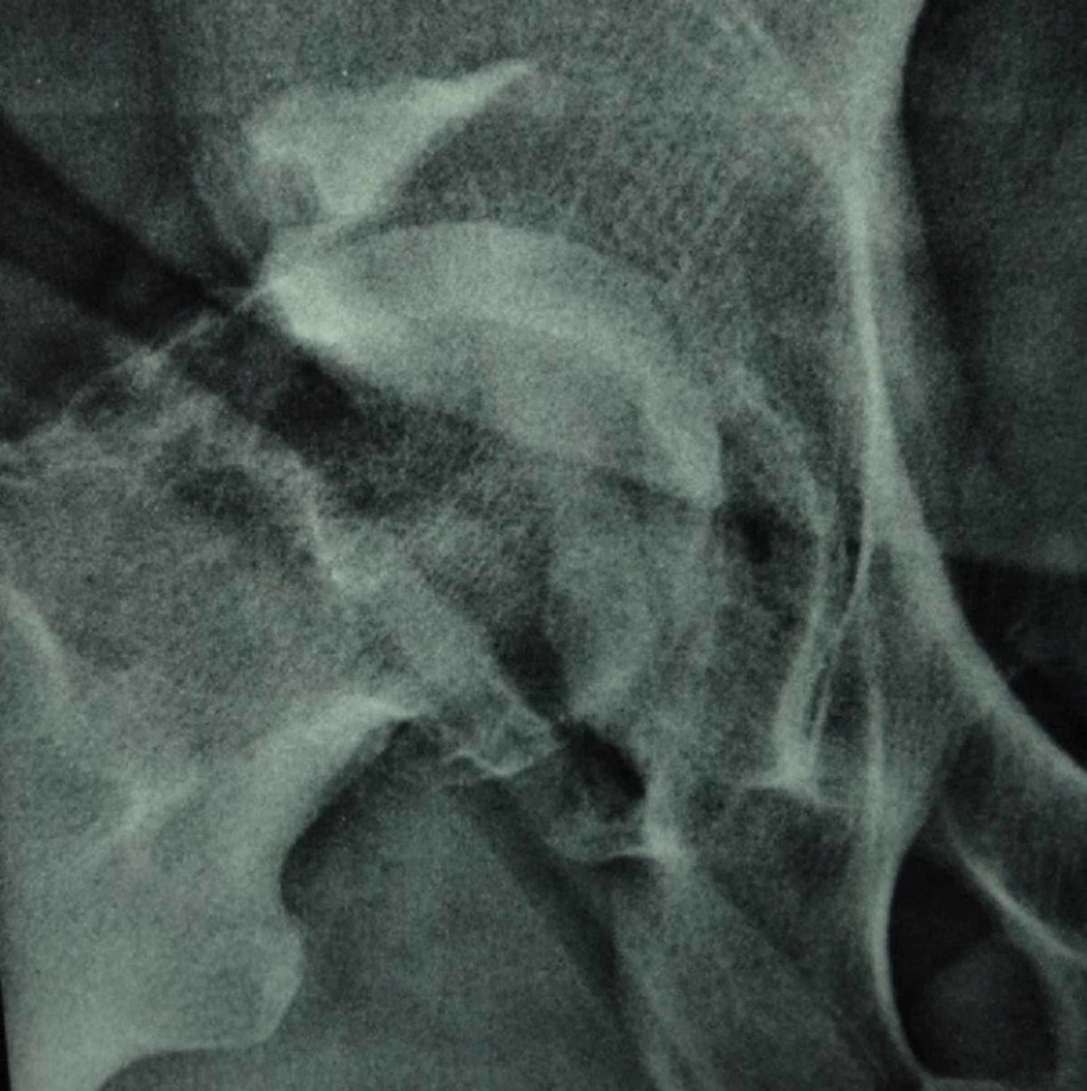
Preoperative X-ray of an 81-year-old patient with an acetabular fracture
